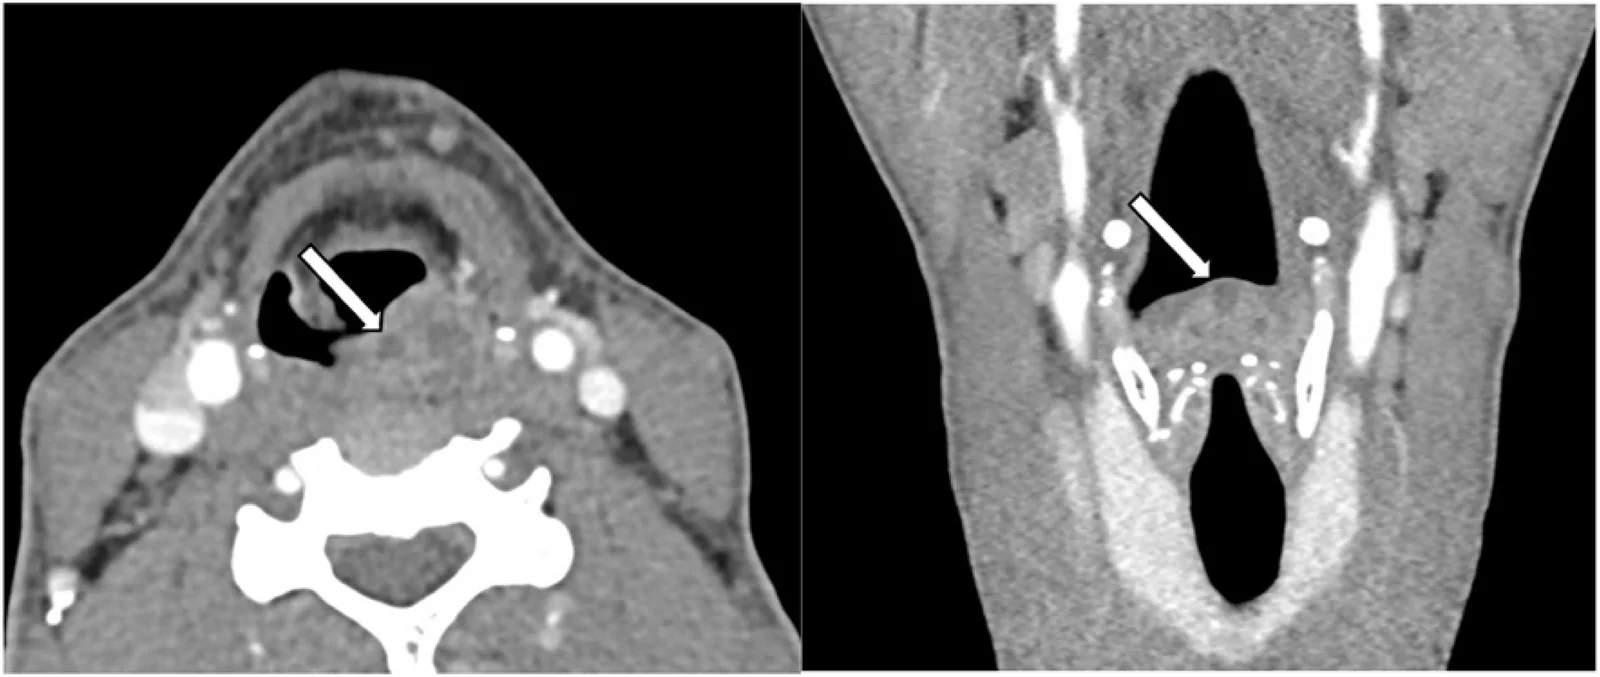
Axial and coronal post-contrast CT images before the first hospitalization demonstrate diffuse thickening and heterogeneous enhancement of the aryepiglottic folds, more pronounced on the left, where a poorly defined oval hypoattenuating area is observed (arrows).
